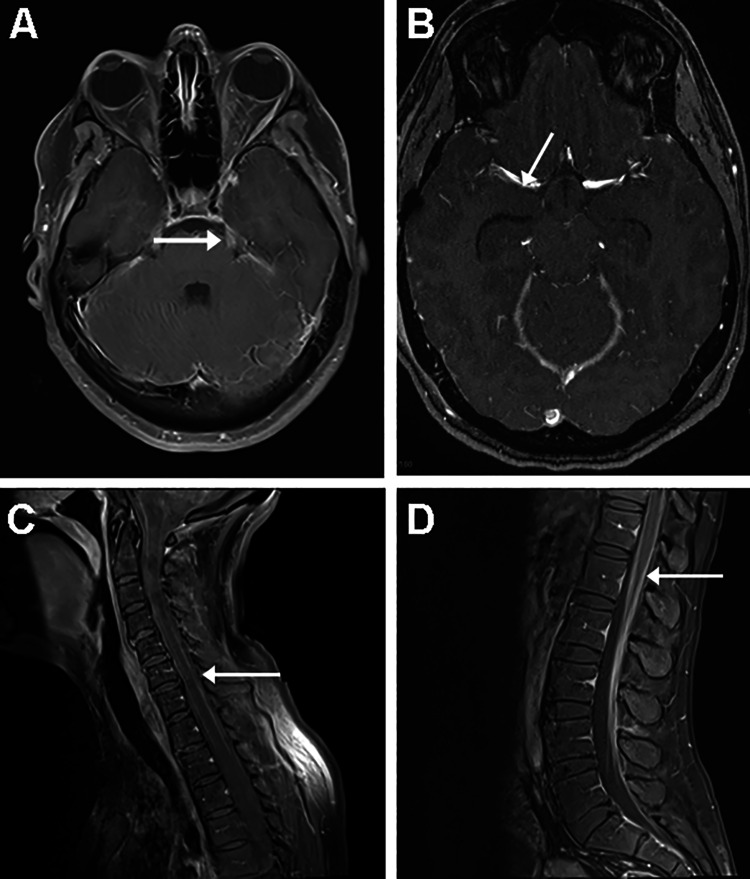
Repeat MRI of the brain Axial T1-weighted postcontrast MRI scans demonstrating (A) leftward midbrain/pontine enhancement and (B) vascular leptomeningeal enhancement around the right middle cerebral artery consistent with LMD. Sagittal postcontrast MRI of the (C) cervical and (D) lumbar spine demonstrating diffuse LMD of the tumor. LMD: Leptomeningeal dissemination
